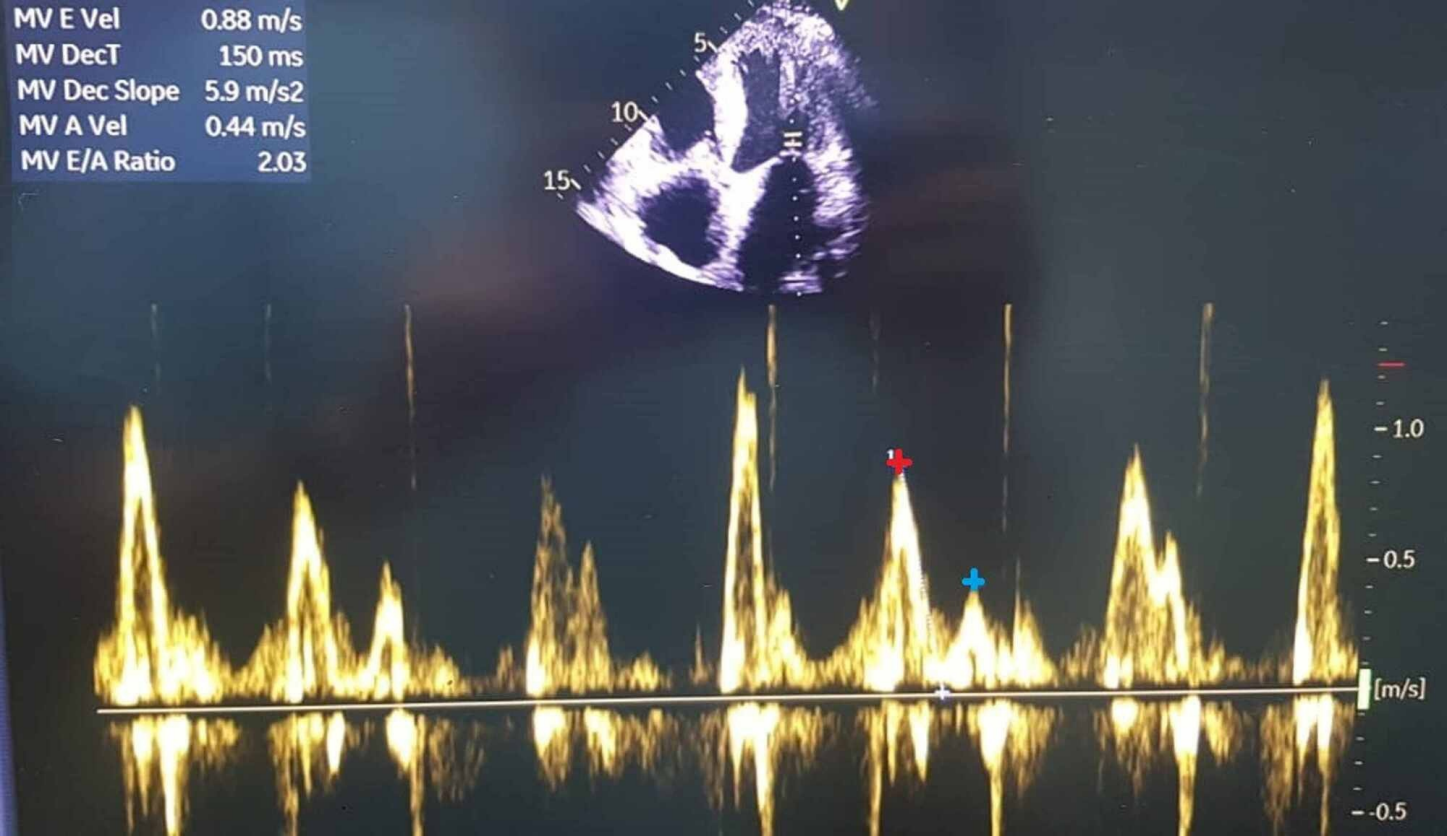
Four chamber view on pulse wave Doppler showing E/A: 2.03 Red + : E; Blue + : A
